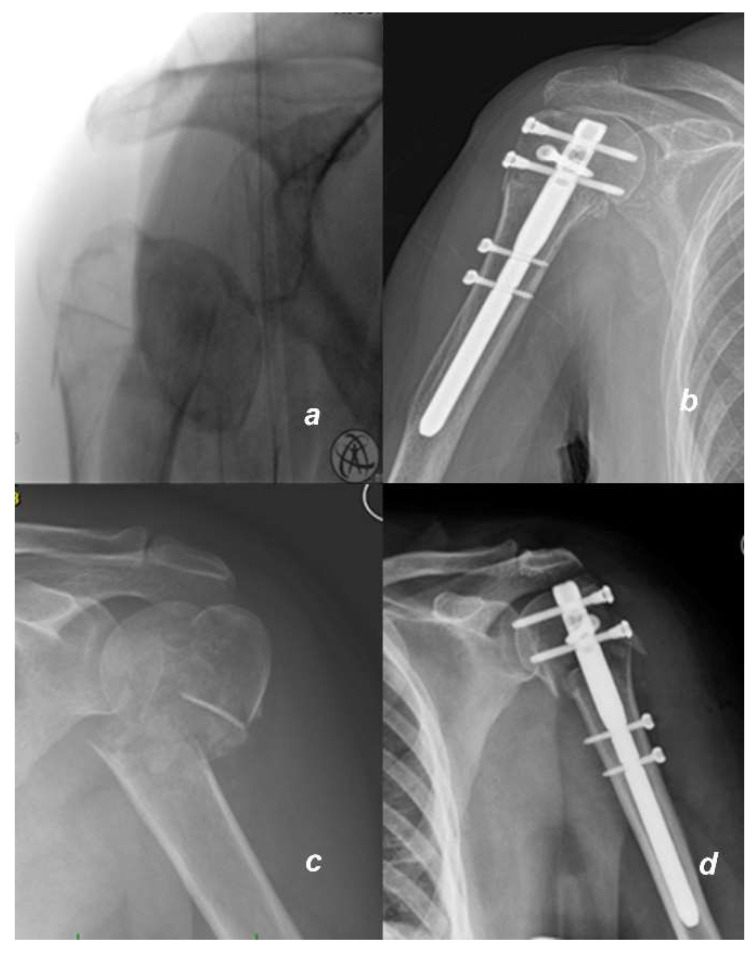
X-rays of fractures and osteosyntheses in two patients: (a) Fracture of proximal humerus in a 57-years-old man with intraarticular fracture 11C1.1 (AO) before and (b) 12 weeks after osteosynthesis with humeral intramedullary nail; as the nail is not protruding, only proximal locking screws would be removed during arthrolysis; (c) a 65-year-old woman with a proximal humerus fracture 11C3.1 before and (d) 6 weeks after osteosynthesis; the nail is protruding. All screws including the nail would be removed during arthrolysis.
